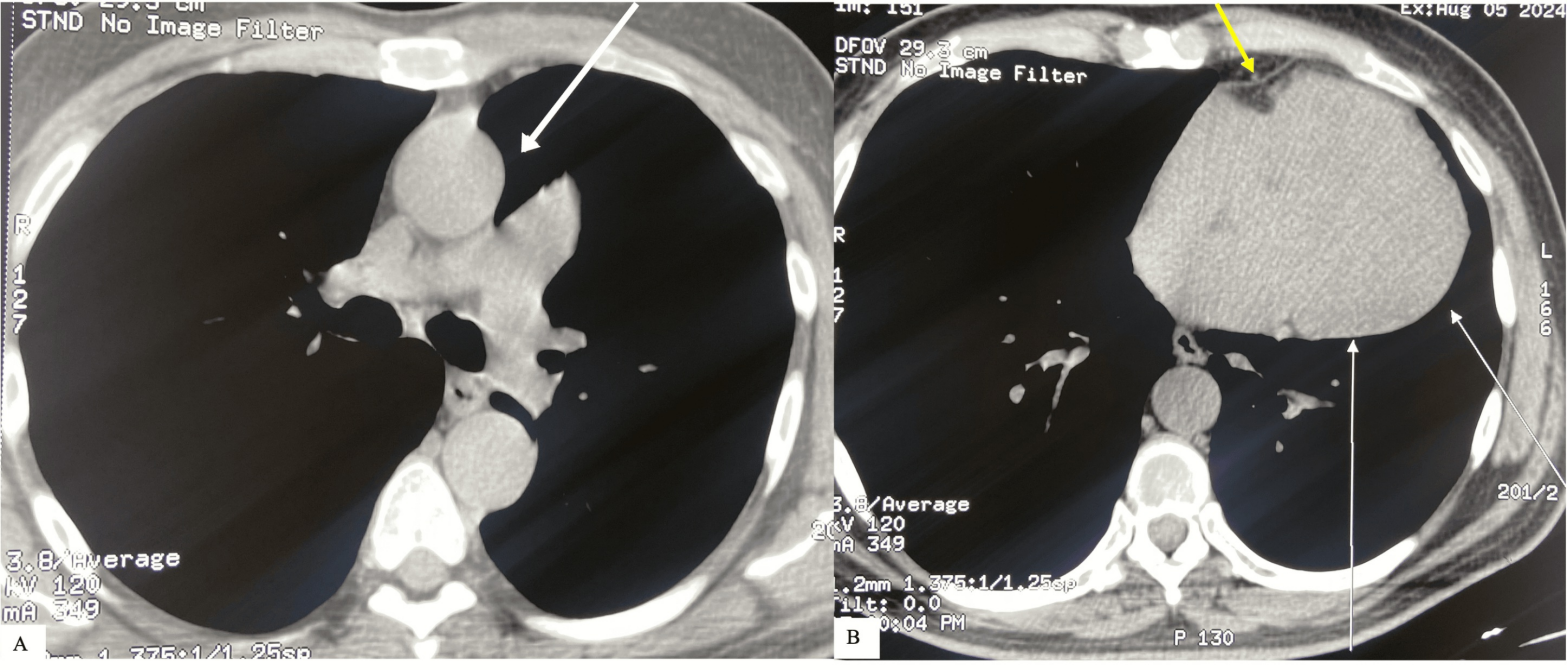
Cardiac CT axial frame images A: Demonstrates interposition of lung tissue between the aortic root and main pulmonary artery (arrow); B: Depicts complete absence of the pericardium along the left ventricular border (white arrows), with pericardium preserved only at the acute margin of the heart (yellow arrow). Also, note the leftward displacement of the heart into the hemithorax.
